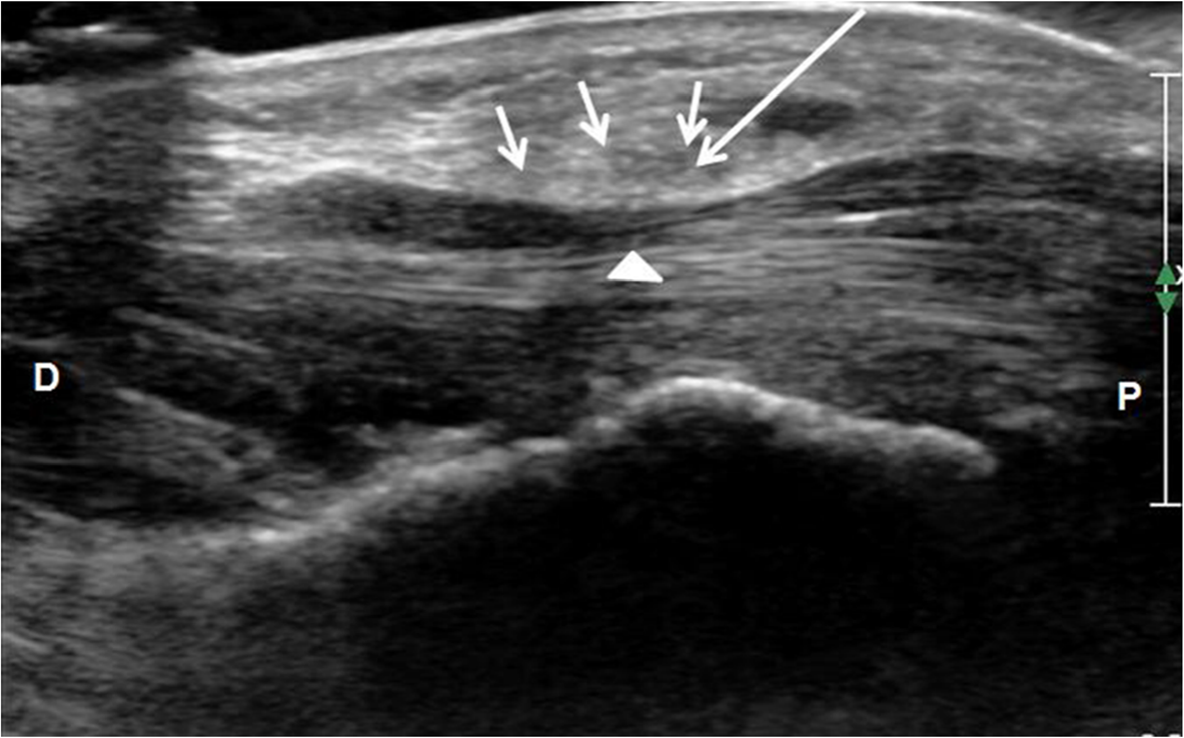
Longitudinal ultrasonic image of carpal tunnel. Long arrow suggests the entry needle route of release. Triangle arrow suggests median nerve compression. Short arrow suggests thickened TCL. *P* proximal end; *D* distal end
